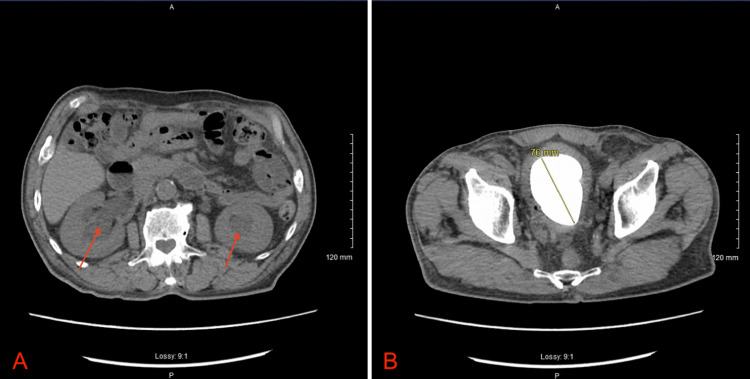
(A) Axial section of computed tomography of the abdomen and pelvis demonstrating bilateral renal hydronephrosis (red arrows). (B) Axial section of computed tomography of the abdomen and pelvis demonstrating a 7.6 cm bladder stone.
